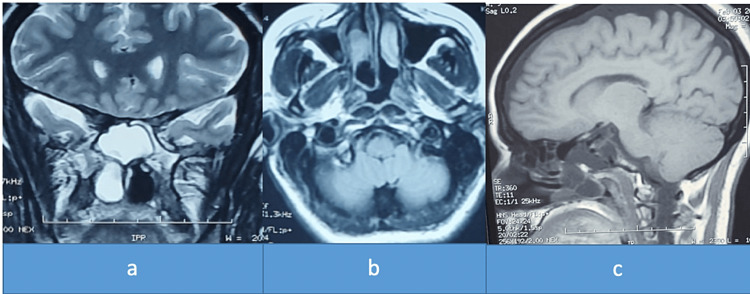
MRI showing the meningocele hyposignal T1 and hypersignal T2 with the same CSF signal in the right nasal cavity
